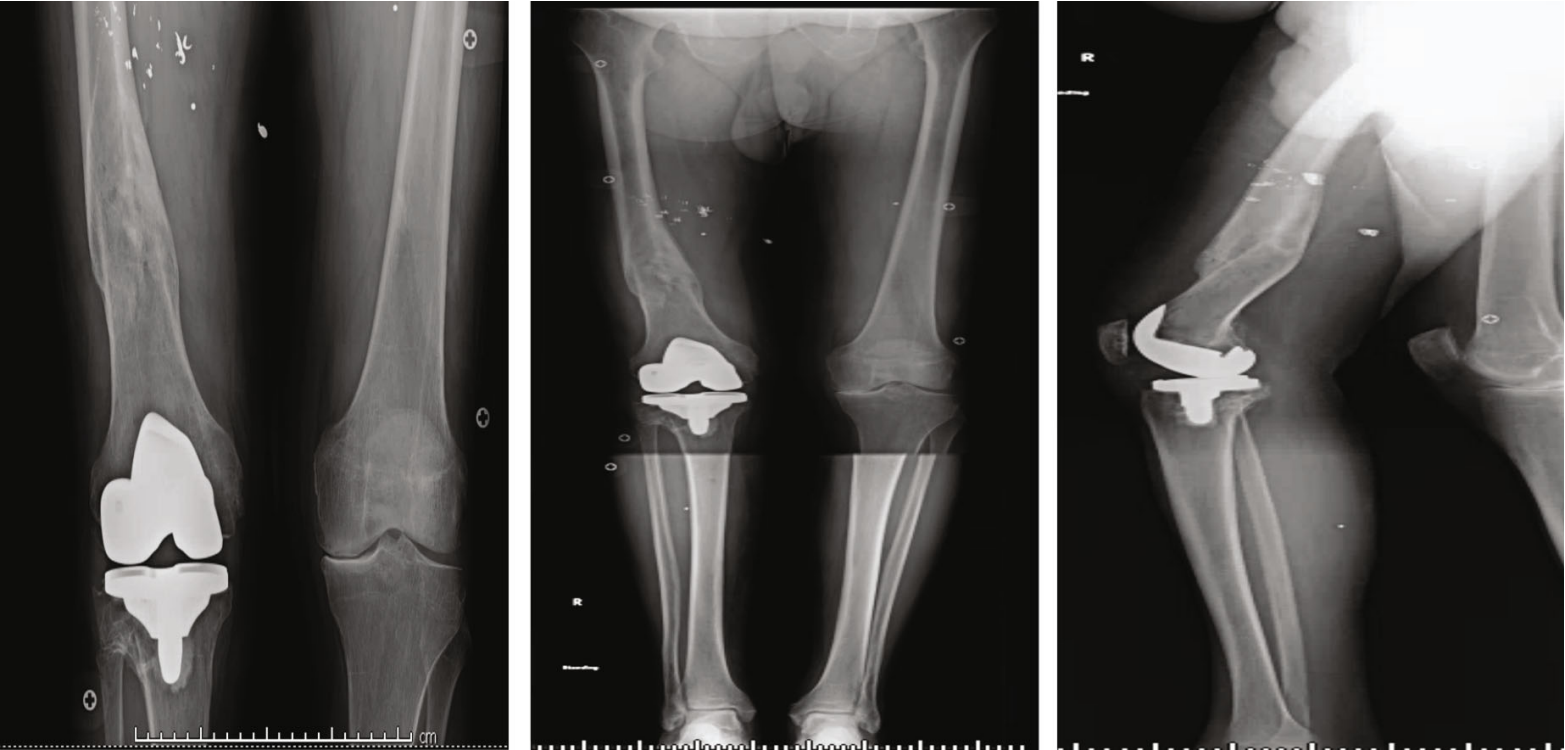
Six-month postoperative X-rays of anteroposterior bilateral knees, bilateral leg length, and lateral leg length flexion.
